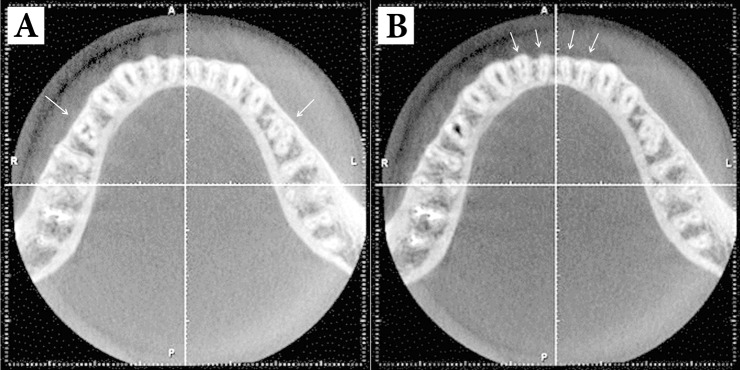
Axial images of CBCT presenting: A: Bilateral three-root mandibular second B: Second canals of all mandibular incisors are visible
